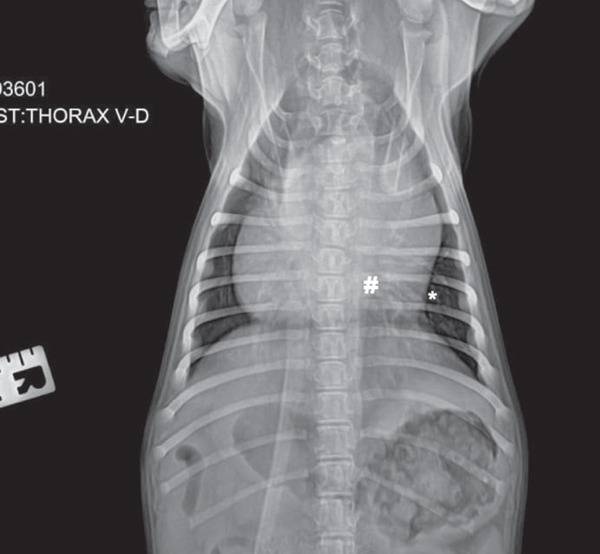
DV chest radiograph. Globular cardiac silhouette (#), interstitial and bronchial pattern (∗), suggestive of generalized cardiomegaly and bronchopneumonia.
